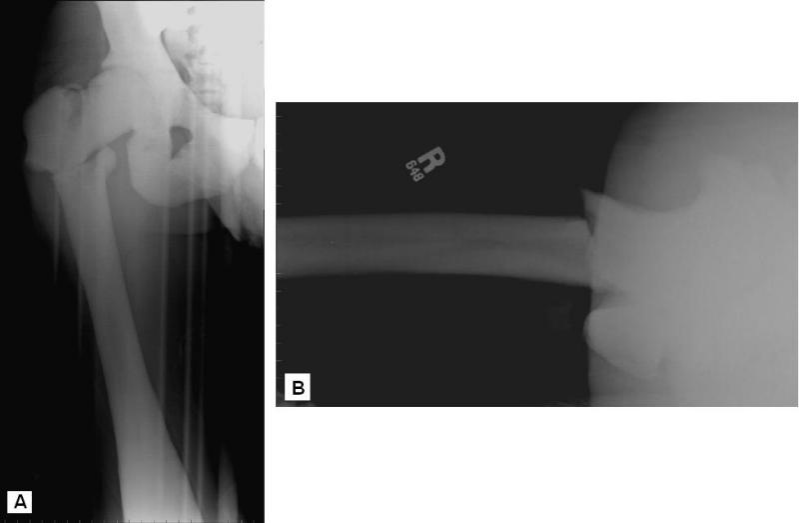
Anteroposterior and lateral radiographs of our patient's right hip at the time of initial presentation to the emergency department.
